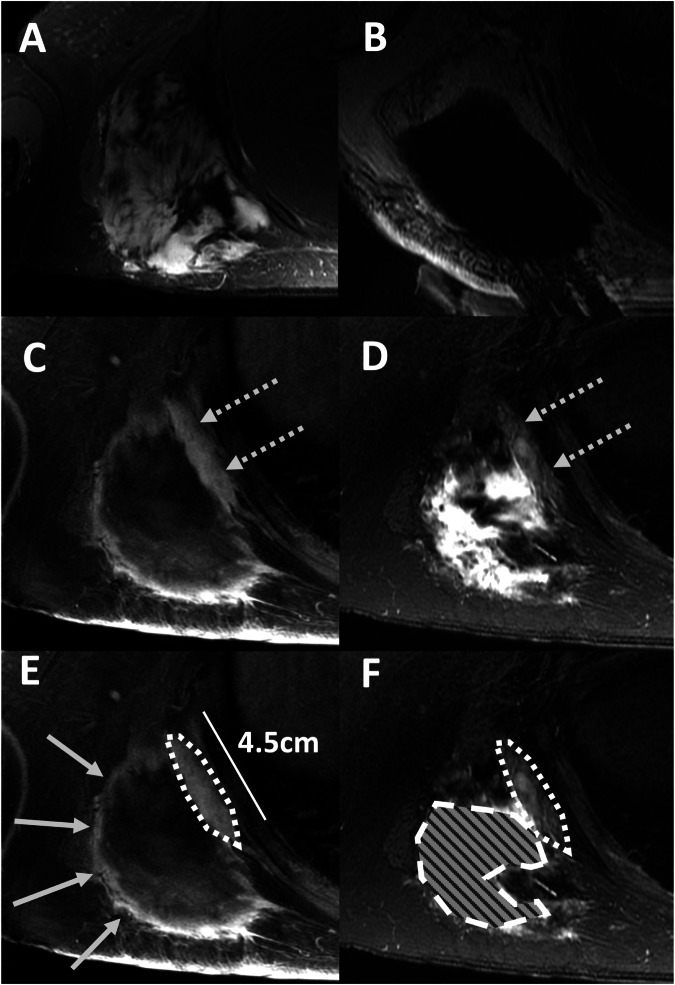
MR-guided cryoablation and post-ablation MRI features in follow-up MRI. A Desmoid tumor in the right shoulder of a 30-year-old female (14 × 9 × 12 cm), causing significant pain and limiting motion of the scapula (axial T1 weighted post-contrast image). B Cryoablation: Treatment of the tumor, which included a pullback and second round of treatment (Axial PD weighted images). C, D Residual disease (dashed arrows) along the chest wall measuring 4.5 cm in follow-up MRI 263 days following cryoablation (axial T1 weighted SPGR post-contrast (C) and axial T2 weighted SSFSE with fat suppression images (D)). E, F Key post-ablation imaging features used in the paper, with contours overlaid on images C and D. The dotted outline shows desmoid tissue that has intermediate T2 signal [+iT2] and enhances [+ENH], but appears linear and not nodular [−NOD]. The dashed outline filled with a pattern shows an example of an area of marked (fluid-like) T2 hyperintense areas that was attributed to post-ablation changes and not considered +iT2 signal. The arrows point to a region that only exhibits +ENH. Examples of nodularity [+NOD] are shown in later figures
